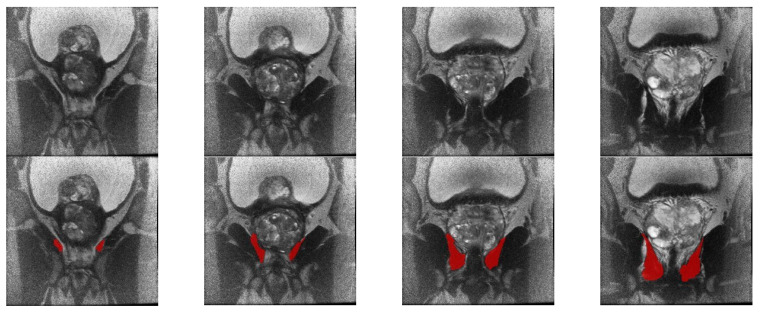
Visual appearance of the levator ani muscle region in the standardized coronal T2-weighted MRI image (top row) and the delineated mask (bottom row) for the region of interest.
